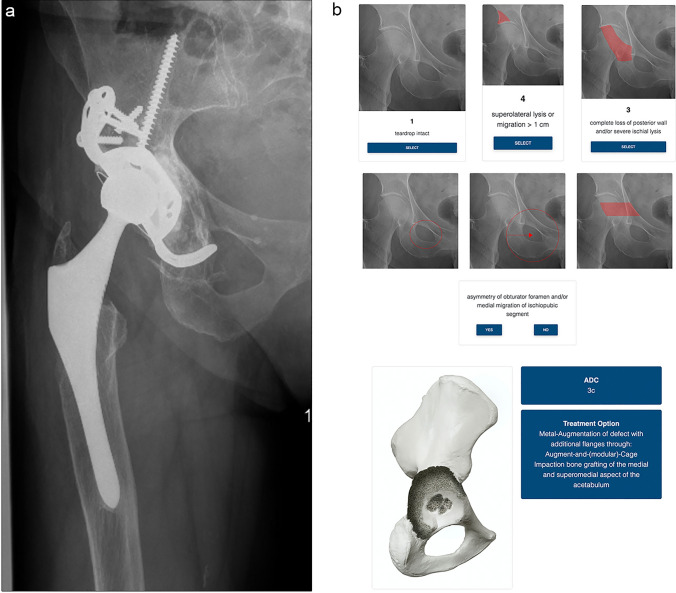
The presentation of the app-guided classification of a pre-operative X-ray of a 67-year-old, female patient with multiple previous operations of the affected hip. a shows the pre-operative AP pelvis view, loosening of the acetabular cage with superolateral migration and consecutive breakage of the screws on the cranial strap; b lists the selected subdivisions within the application: medial: the teardrop appears intact due to the superolateral migration; superior: the superolateral migration ensures a superior defect, removal of the fractured screws and bony debridement will further increase defect size, a superior defect of > 1 cm is to be expected; posterior: with the implant still in place radiopaque material limits the visualization of the posterior acetabular wall, cloudy osteolysis of the visible aspects indicate the presents of a bony defect, which should be selected accordingly. To further evaluate the posterior acetabular wall a CT scan can be helpful; pelvic discontinuity: the presented X-ray displays no indicators to suspect pelvic discontinuity. an ADC 3C defect with a treatment option is displayed
